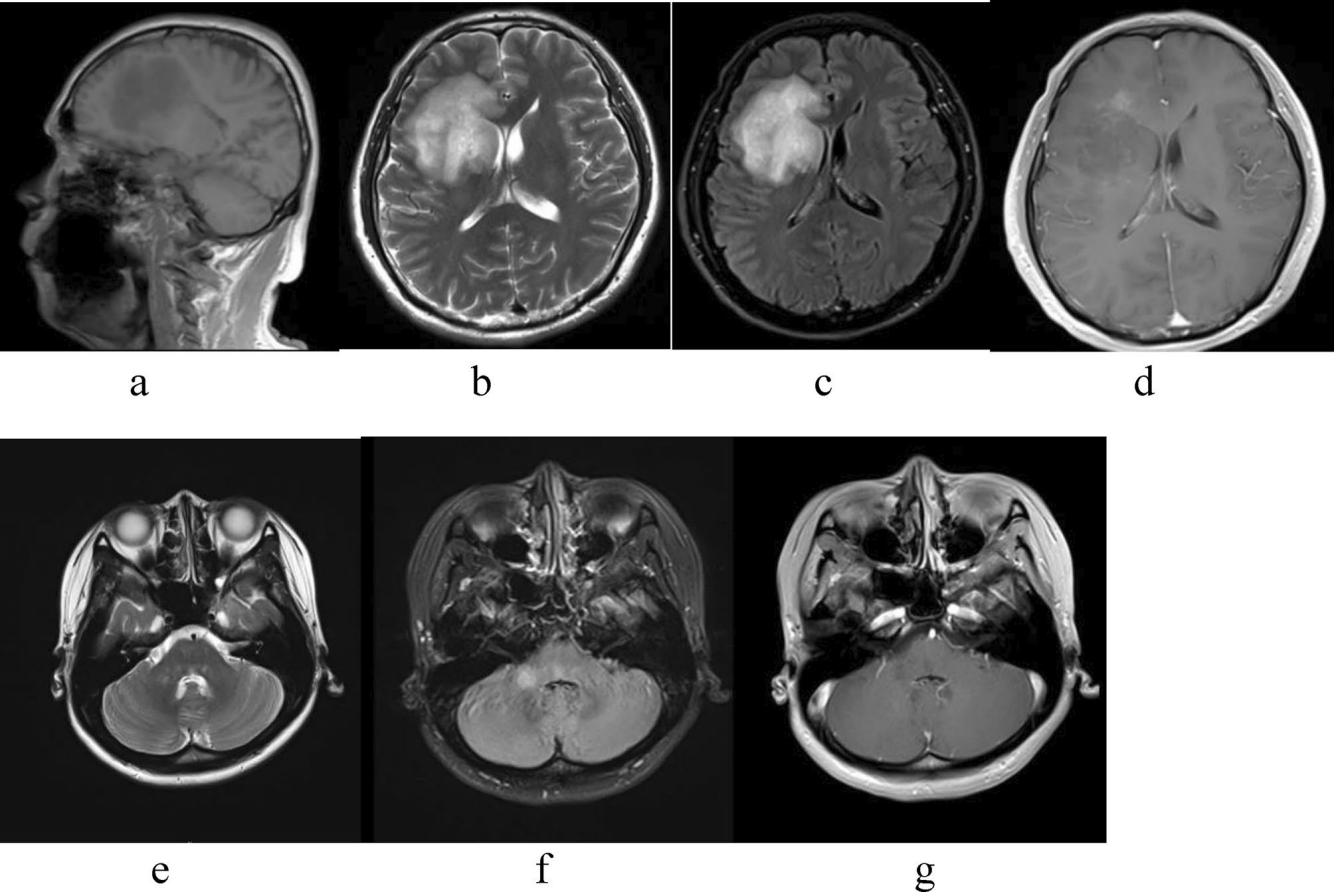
Male, 57 years old, dizziness and memory loss for more than 1 month (a–d). The patient relapse after 1 year, weakness of left limbs and seizures (e–g). (a) T1 sagittal position: right frontal lobe, corpus callosum, basal ganglia, and periventricular long T1 signal; (b) T2 axial position: right frontal lobe, corpus callosum, basal ganglia, and periventricular long T2 signal; (c) Flair axial position: right frontal lobe, corpus callosum, basal ganglia, and periventricular high signal; (d) enhanced MRI axial position: patchy enhancement. The patient relapse after 1 year (e–g). (e) T2 axial position: right brachium pontis long T2 signal; (f) Flair axial position: right brachium pontis high signal; (g) enhanced MRI axial position: none enhancement.
